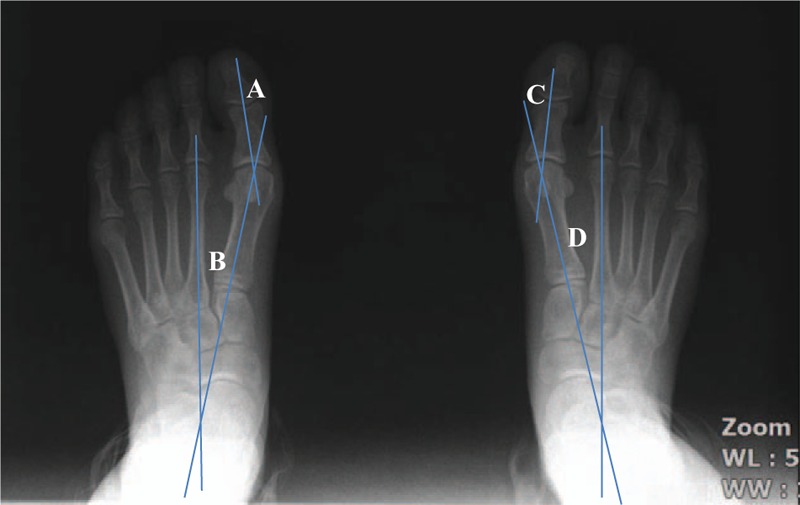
Initial weight bearing radiographic view of both feet (A, left hallux valgus angle; B, left intermetatarsal angle; C, right hallux valgus angle; D, right intermetatarsal angle).
